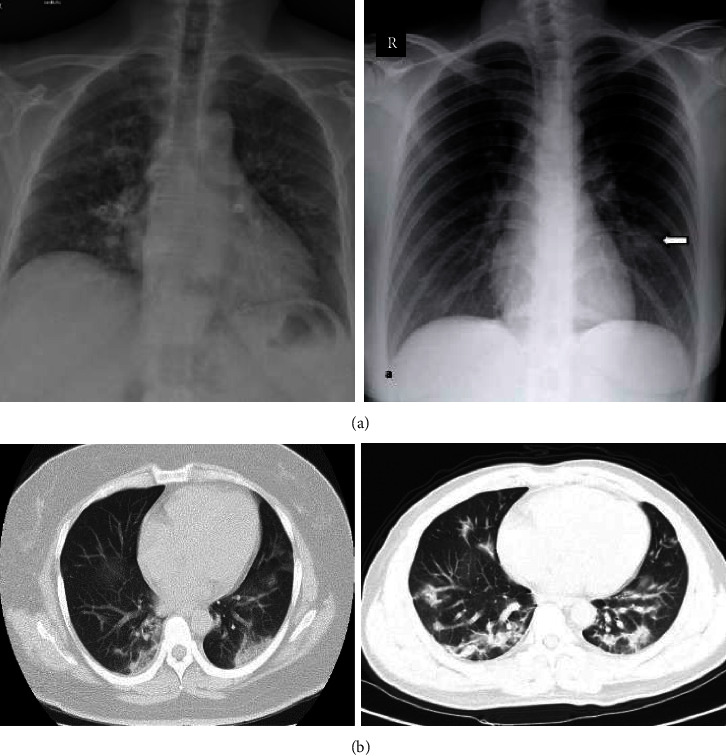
(a) Sample X-ray images of COVID-19-affected lungs [2]. (b) Sample CT scan images of COVID-19 patients [3].
